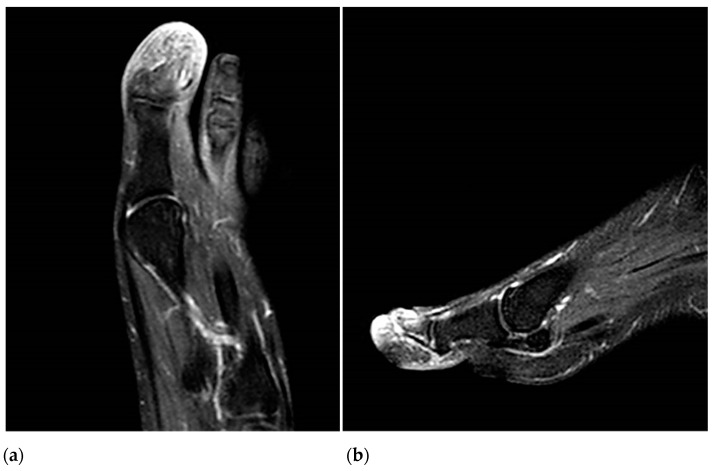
T2-weighted coronal (a) and axial (b) MRI images show bone edema in the distal phalanx with inflammatory changes in the soft tissues that are more intense in the subungual region.
